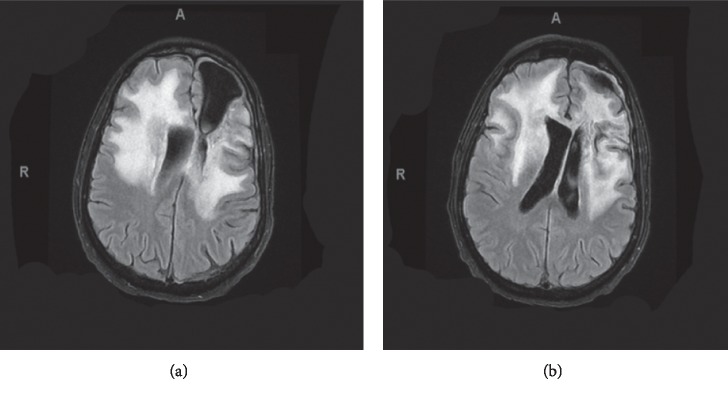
Pretherapy T2 FLAIR, March 2018. (a) and (b) demonstrating significant cerebral edema and midline shift.
